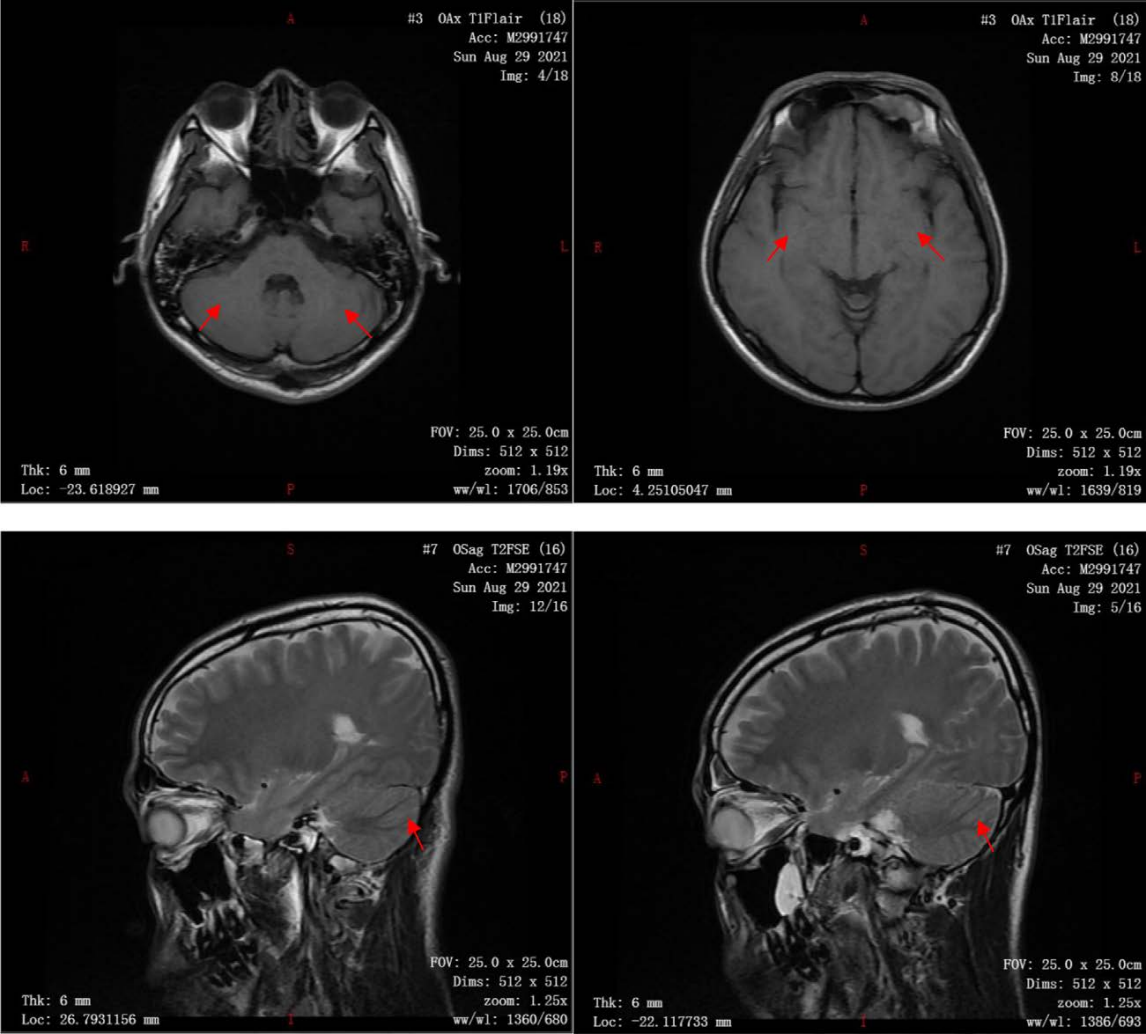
After treatment and re-examination, asymmetrical distribution of patchy slightly longer T2 signal was seen in the hippocampal region bilaterally with better than before, slightly higher signal in the pressurized water and diffusion-weighted imaging (DWI) sequence.
